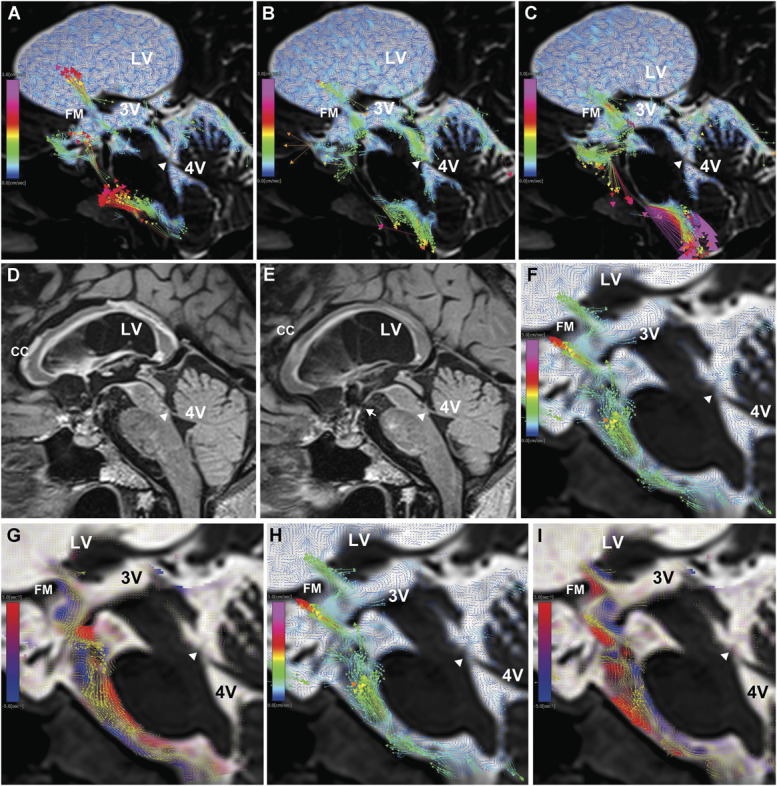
Obstructive hydrocephalus (case 1). A-C, Sagittal views of 4D flow MRI before treatment. The white arrowhead indicates the obstructed site at the lower end of the cerebral aqueduct, and the colored arrows represent velocity vectors. The FM exhibited the highest bidirectional CSF flow velocity, whereas slow bidirectional flow was also observed within the obstructed cerebral aqueduct. D, Sagittal view of 3D FLAIR image taken 4 months after VPS. The CC exhibited widespread high signal intensity across its entire length, as did the upper midbrain and the third ventricular floor. E and F, Sagittal views of 4D flow MRI after ETV. Rapid CSF flow was observed from the FM into the 3V and through the fenestration in the third ventricular floor (white arrow) into the interpeduncular cistern. During a single cardiac cycle, the flow reversed, showing rapid CSF movement from the interpeduncular cistern into the 3V and from the 3V to the LV through the FM. G, Sagittal view of 3D FLAIR image after ETV. Compared with pre-ETV imaging D, the widespread high signal intensity in the CC, upper midbrain, and third ventricular floor had improved. H and I, Sagittal views of 4D flow MRI after ETV. Clockwise vortices are shown in blue, counterclockwise vortices in red, with yellow velocity vectors overlaid. A clockwise vortex was observed in the anterior part of the 3V. 3D, 3-dimensional; 3V, third ventricle; 4D, 4-dimensional; 4V, fourth ventricle; CC, corpus callosum; CSF, cerebrospinal fluid; ETV, endoscopic third ventriculostomy; FLAIR, fluid-attenuated inversion-recovery; FM, foramen of Monro; LV, lateral ventricle; VPS, ventriculoperitoneal shunting.
